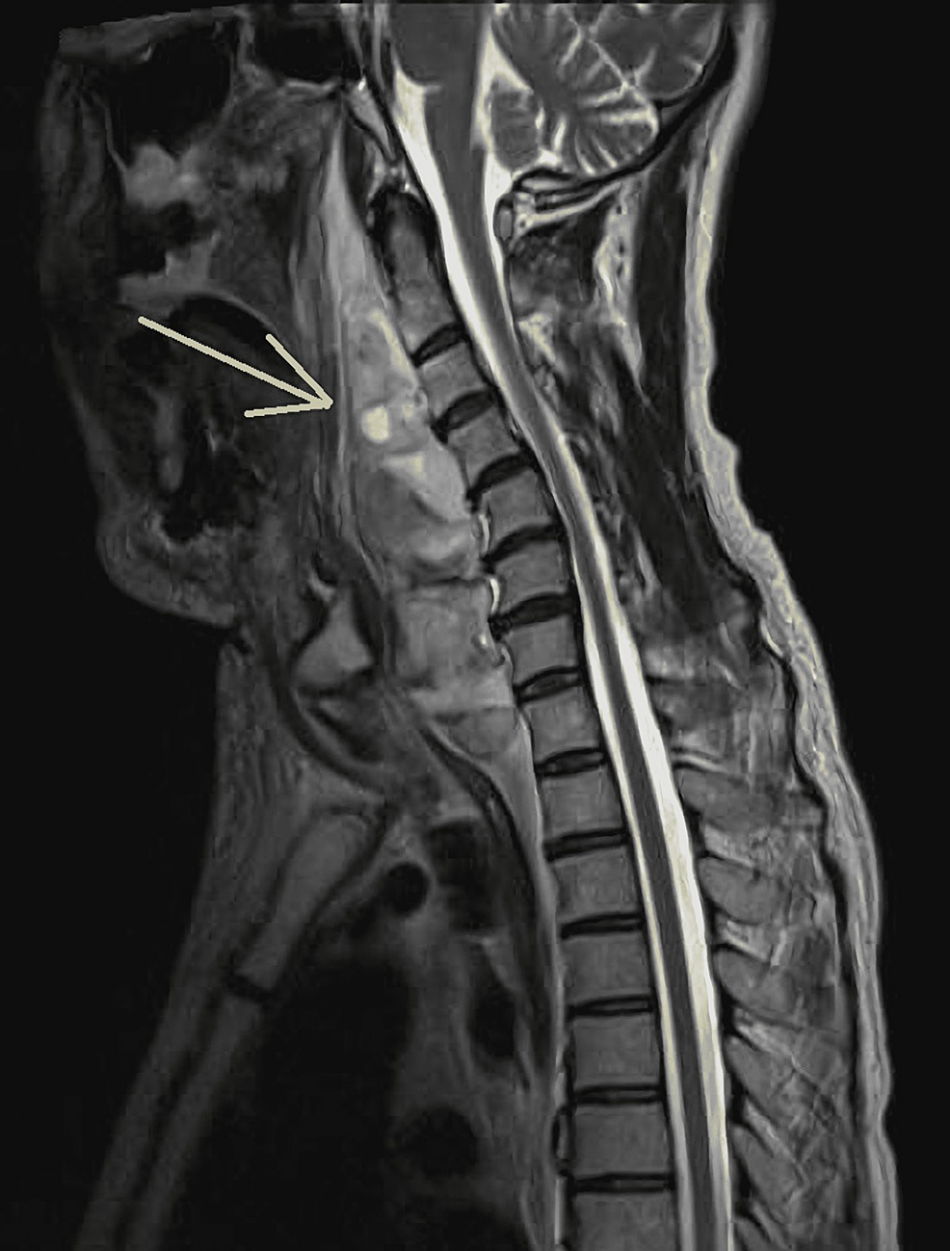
Cervical spine MRI at initial examination (sagittal) No obvious cervical fracture is seen. The arrow indicates a high-density area, which extends over the anterior cervical spine and is thought to be a hematoma.
